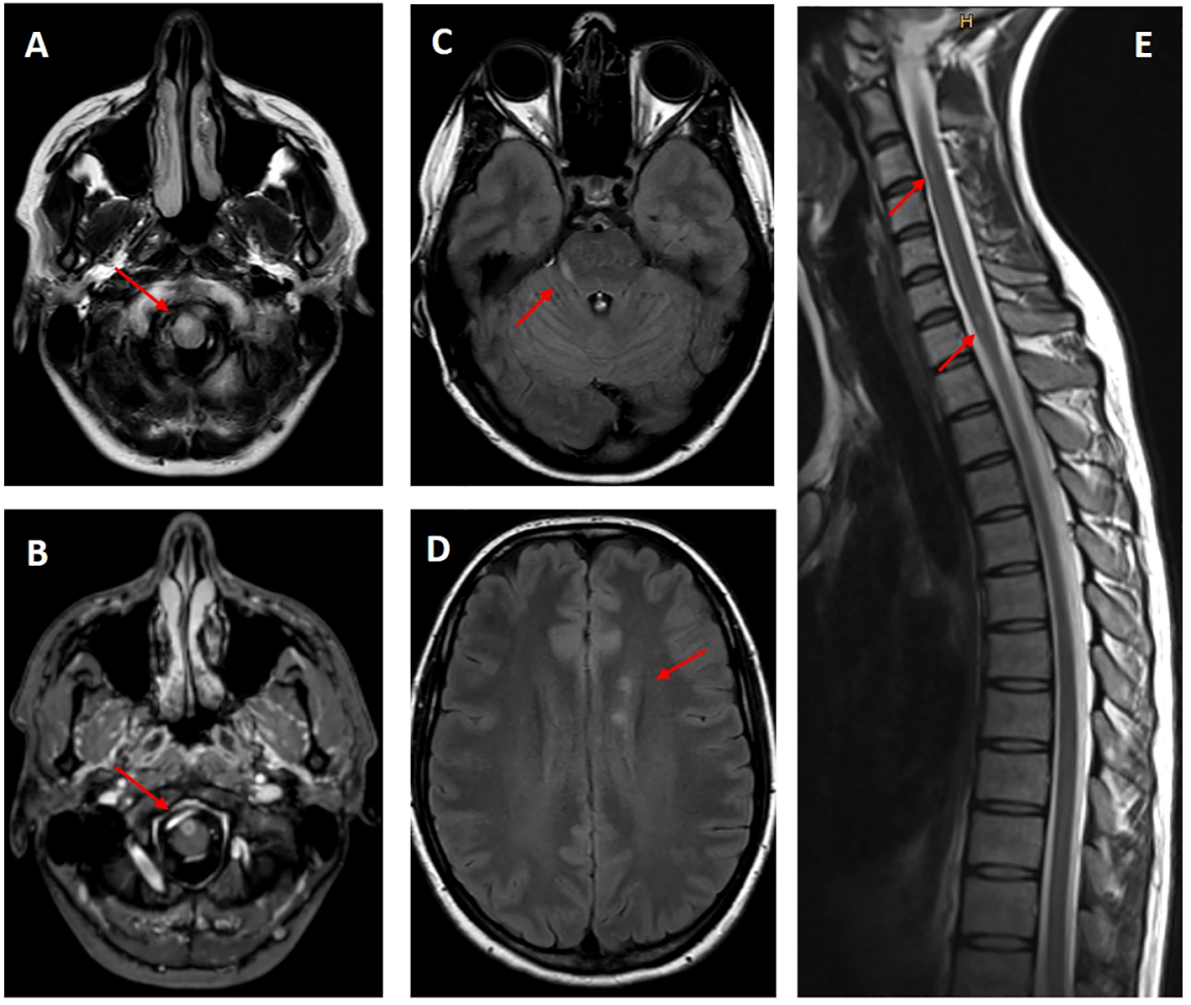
MRI of brain and cervical spine, MS presentation. New T2-hyperintense lesions are seen, amongst others, in the right paramedian medulla oblongata (A), in the right inferior cerebellar peduncle (C), in the periventricular white matter (D) and in the cervical spinal cord (E). Contrast enhancement is seen, amongst others, with the lesion in the right paramedian medulla oblongata (B).
